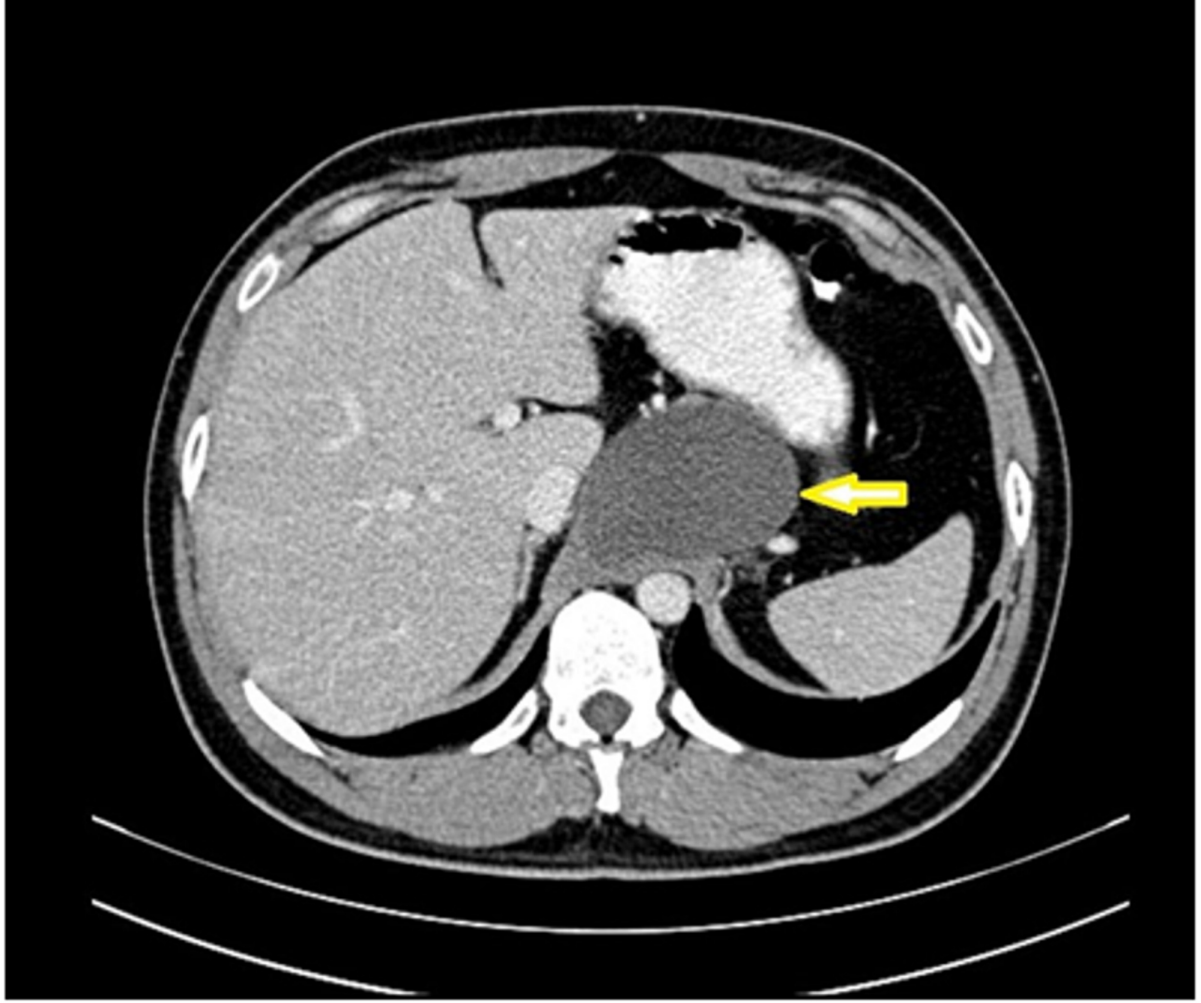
A cut section from contrast-enhanced CT whole abdomen showing the location of the cyst (arrowhead)
